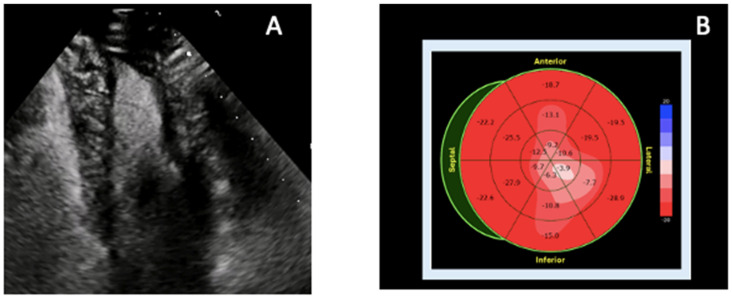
Echocardiographic features of atypical apical involvement in cardiac amyloidosis. In cardiac amyloidosis, apical thickening may mimic ApHCM or focal fibrosis, but multimodality echocardiographic assessment—particularly contrast TTE combined with strain analysis—reveals characteristic patterns that help differentiate infiltrative disease from other apical pathologies. (A) Contrast-enhanced TTE (apical two-chamber view) demonstrates improved endocardial border delineation and increased biventricular wall thickness with a granular sparkling myocardial texture, supportive of atypical apical amyloid infiltration. (B) Speckle-tracking-derived bull’s-eye plot shows disproportionately reduced apical longitudinal strain (“reverse apical sparing”), a deformation pattern associated with apical AL amyloidosis and distinct from the classic RELAPS pattern of ATTR. The combination of granular apical texture, contrast-enhanced visualization, and apex-predominant strain reduction differentiates amyloidosis from ApHCM (where apical strain is typically preserved or only mildly reduced), ischemic scarring (territorial pattern), and LVNC (deep perfused recesses rather than homogeneous thickening). Original images from our institution (single patient).
